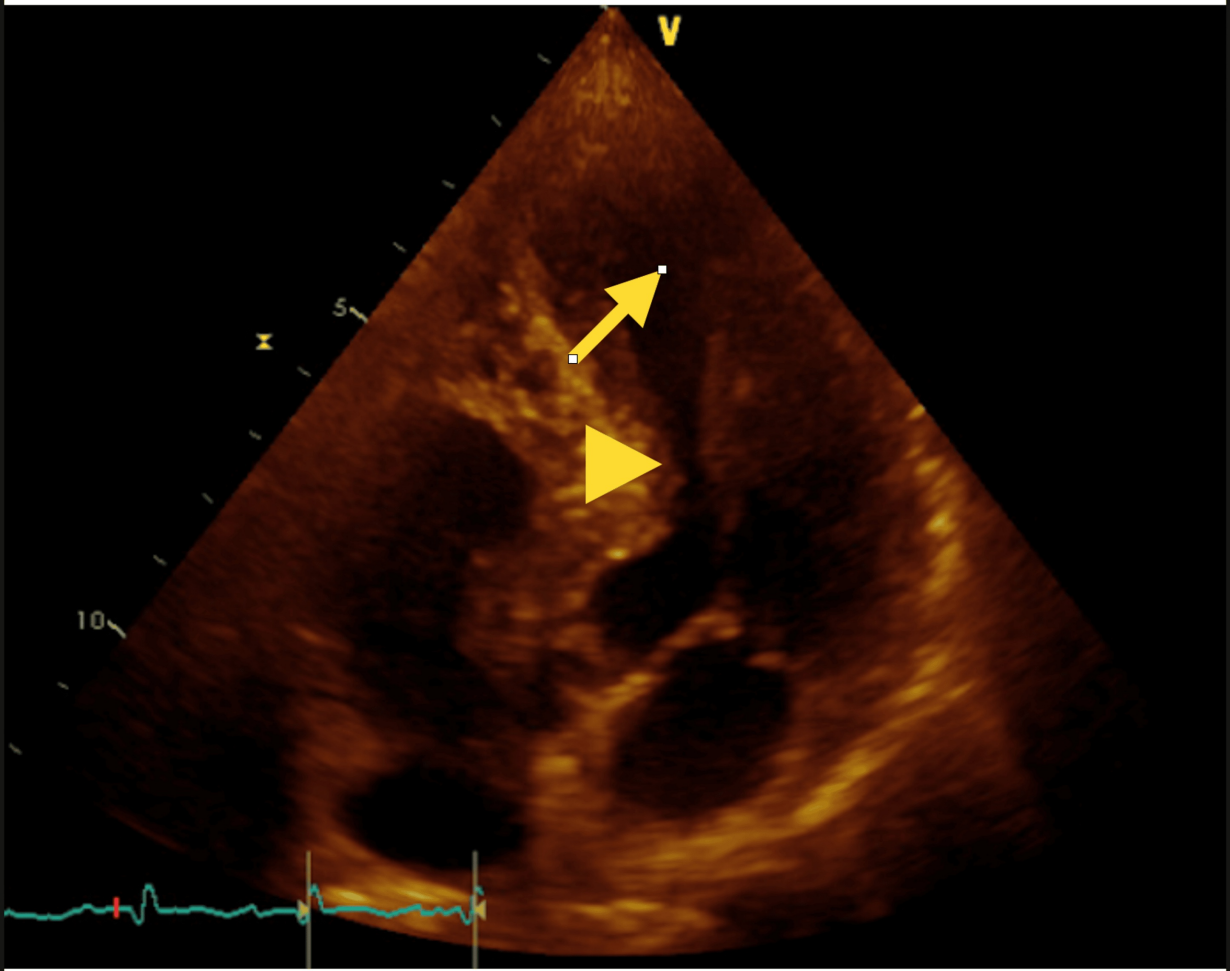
Apical four-chamber view demonstrating classic features of Takotsubo cardiomyopathy, with marked apical ballooning (arrow) and basal hyperkinesis (arrowhead).
